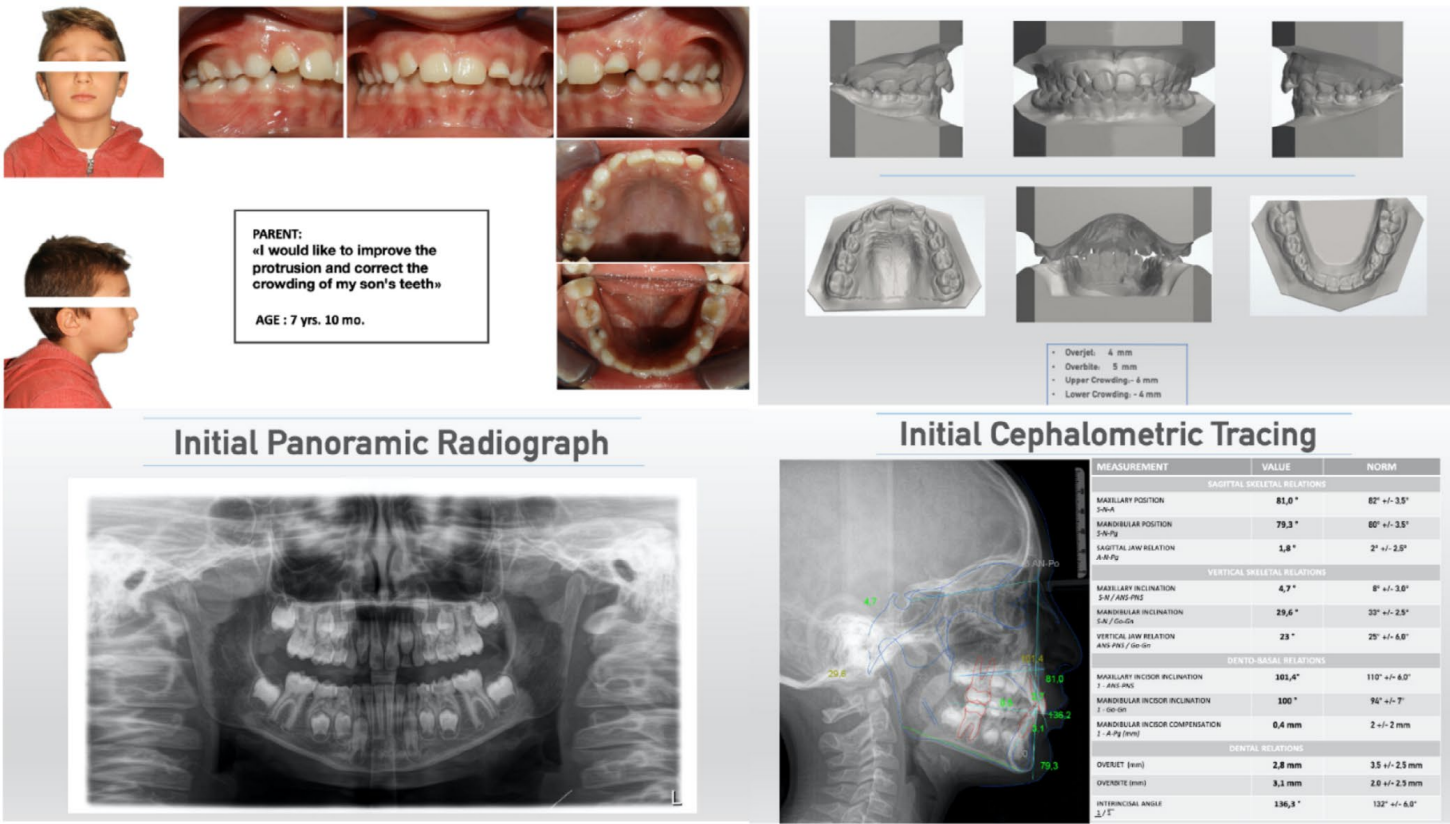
(Upper left) Age, reason for visit, anonymized extra‐oral photos, intra‐oral photos. (Upper right) Digital arch models and overjet value (OJ), overbite value (OB) and upper and lower dental arch crowding. (Lower left) initial orthopantomography. (Lower right) lateral teleradiograph with EBO cephalometric analysis.
